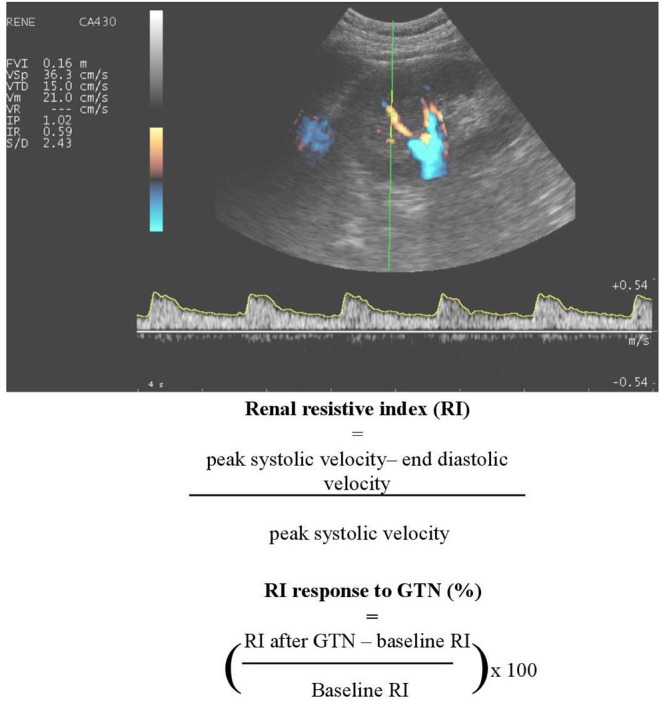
An example of renal Doppler sonography. A number of variables expressing flow pulsatility and vascular resistance can be determined, including renal resistive index (RI) and dynamic RI.
